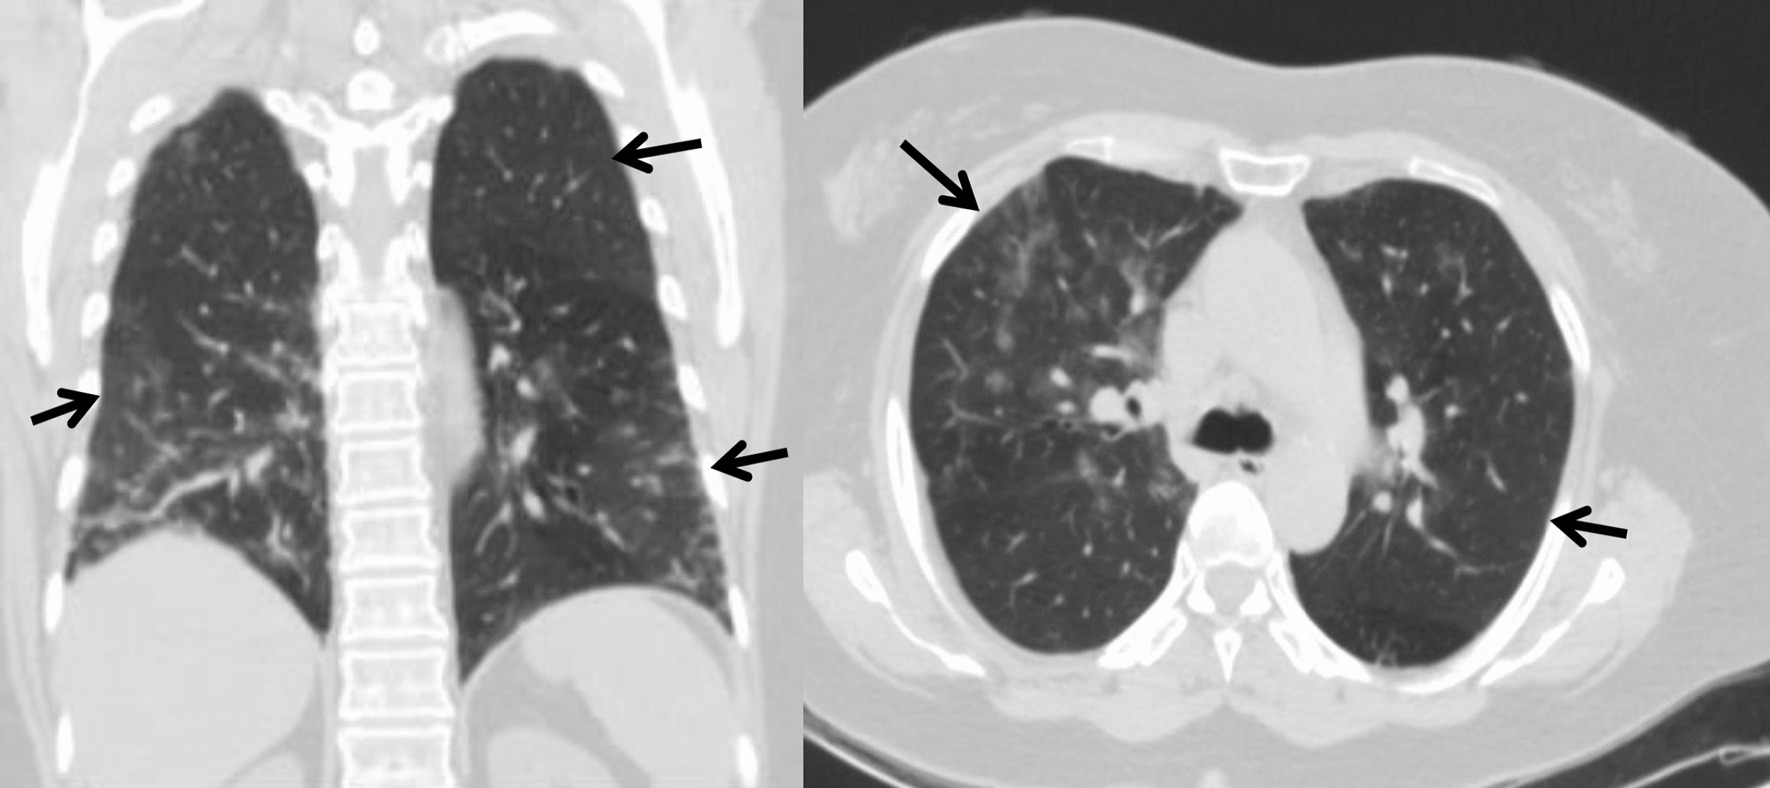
Nonenhanced chest computed tomography images of the patient on day 8 of illness. The computed tomography scans showed bilateral, patchy, ground-glass opacities (arrows)
